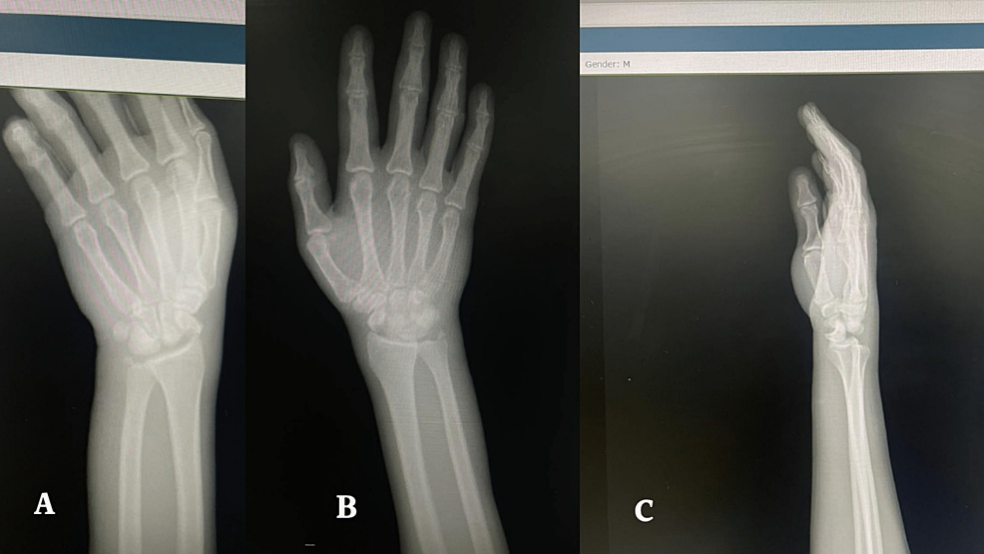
Postero-anterior (A), antero-posterior (B) and lateral views of the right wrist x-ray showing a lunate volar dislocation and scaphoid fracture
